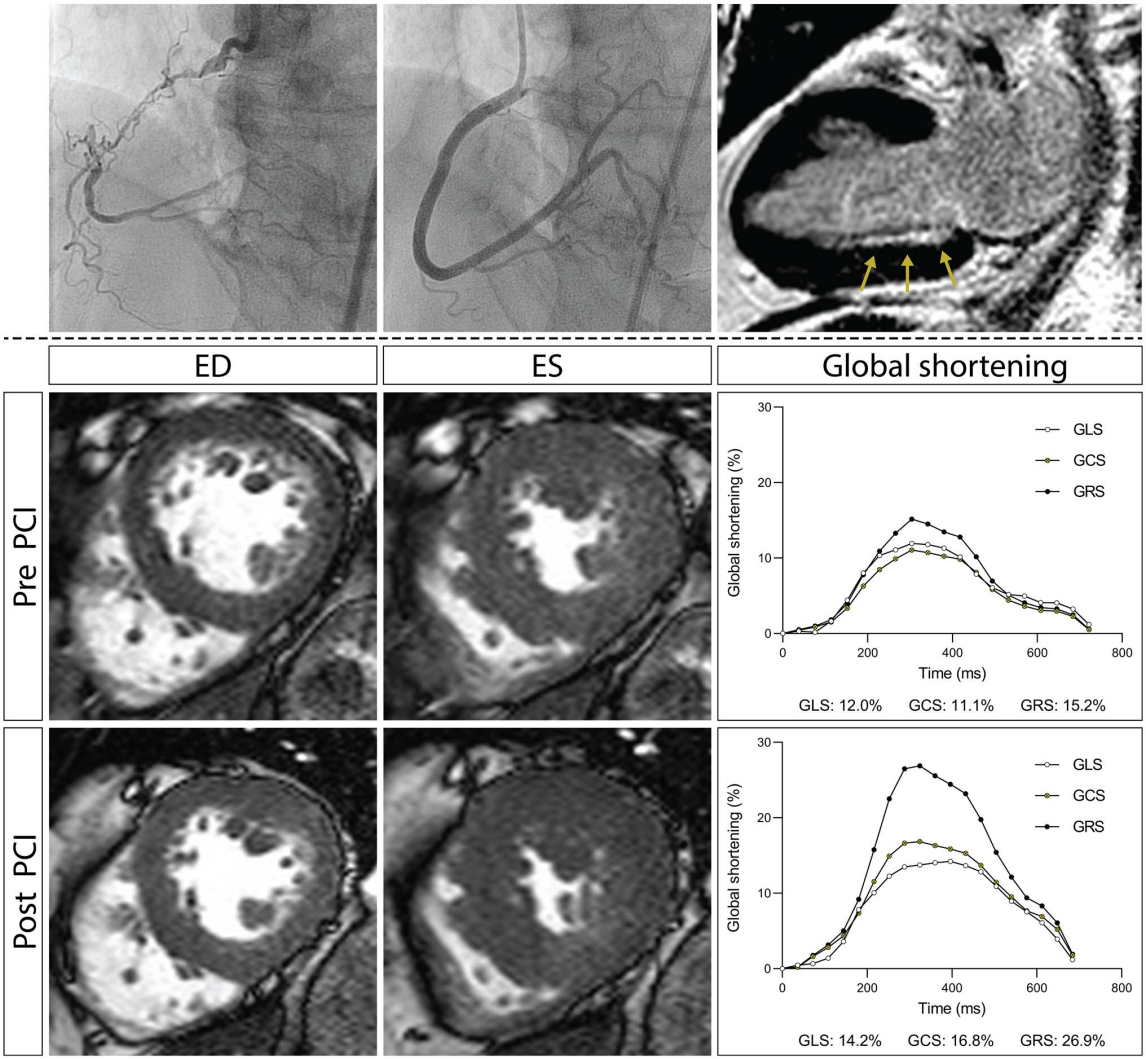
Case example of a patient with a CTO of the RCA. The RCA is proximally occluded and the distal coronary segments are filled through collaterals (top row, left). CMR demonstrates subendocardial hyperenhancement (top row, right, arrows) and regional wall motion abnormalities (middle row, left and center) of the inferior wall. Global longitudinal, circumferential and radial strain are all impaired (middle row, right). Successful restoration of coronary patency (top, middle) results in marked improvements of global myocardial shortening (bottom row). *CMR* cardiac magnetic resonance imaging, *CTO* coronary chronic total occlusion, *ED* end-diastole, *ES* end-systole, *GCS* global circumferential shortening, *GLS* global longitudinal shortening, *GRS* global radial shortening, *PCI* percutaneous coronary intervention, *RCA* right coronary artery
